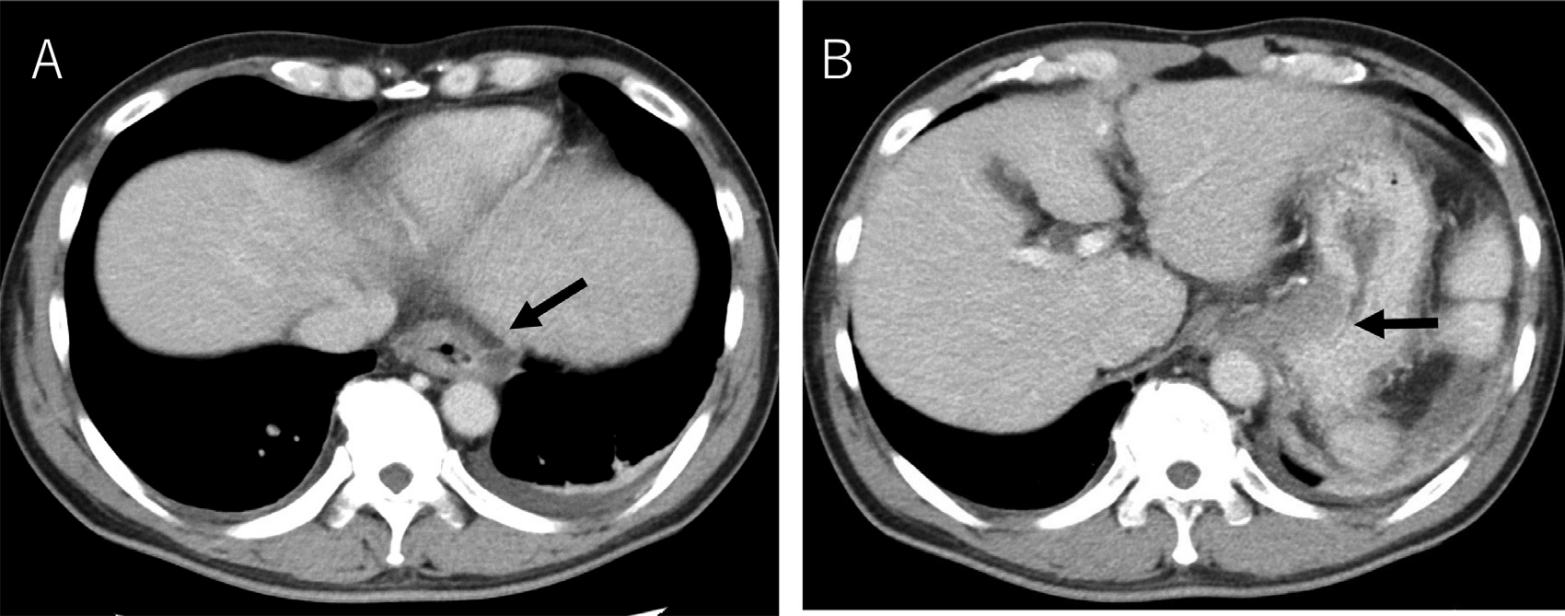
CE-CT image after stepwise PSE and EISML. (A) CE-CT image shows esophageal variceal thrombus formation (black arrow). (B) CE-CT image shows gastric variceal thrombus formation at the cardia. EISML, endoscopic injection sclerotherapy with multiple ligations; PSE, partial splenic embolization.
